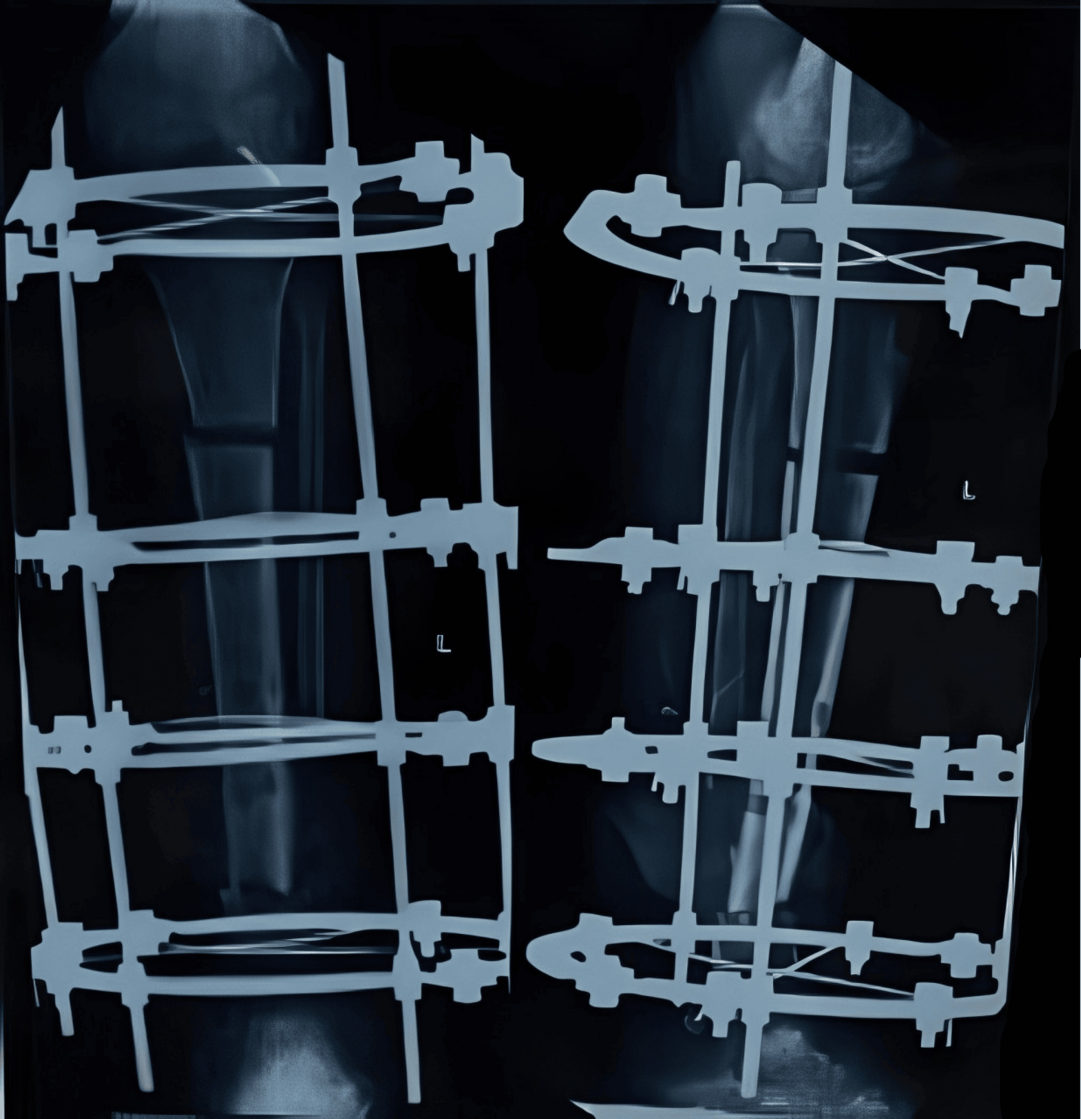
X-ray after one week of distraction at the proximal tibia
